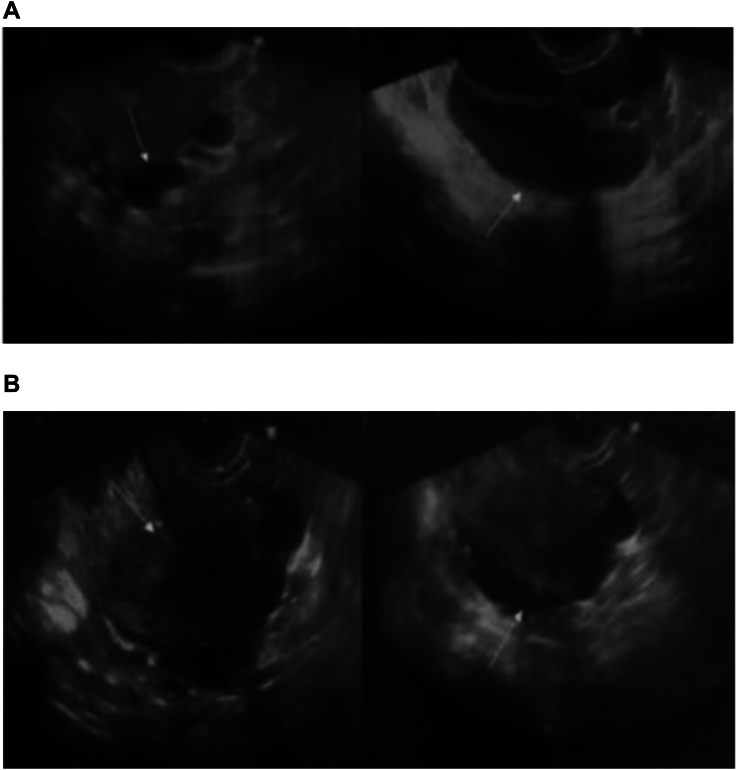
Transvaginal ultrasonography revealed a well-defined, round, multicystic mass measuring 6 cm, characterized by homogeneous cystic contents situated in the cul-de-sac. B: Multiple anechoic cystic formations were observed surrounding the fundus of the uterus.
